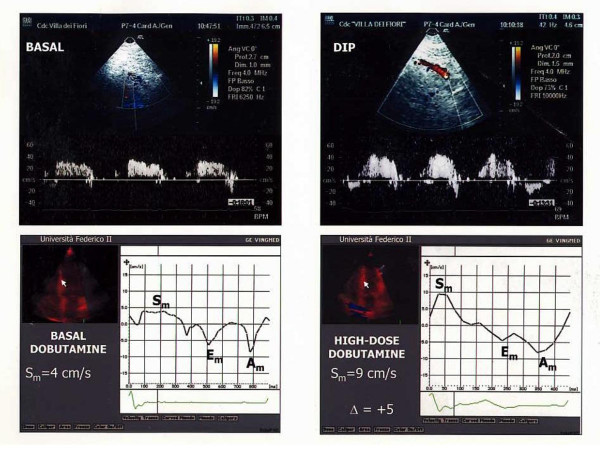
CFR and septal S_m _peak velocity at high-dose dobutamine in a patient with SPECT derived perfusion defect. The upper panels display a reduced CFR. In the lower panels, the increase of septal S_m _from baseline to high-dose dobutamine is low.
